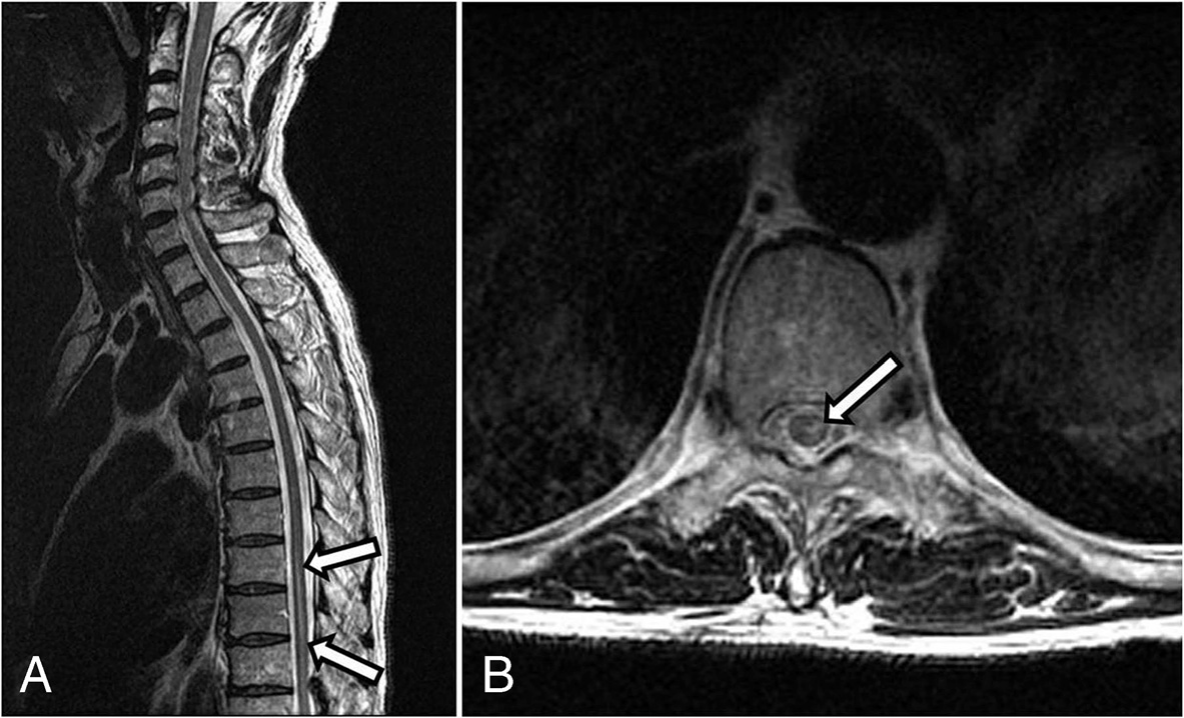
Axial (A) and coronal (B) T2-weighted magnetic resonance images show increased signal intensity (arrows) in the anterior spinal cord at the T8-10 levels.
